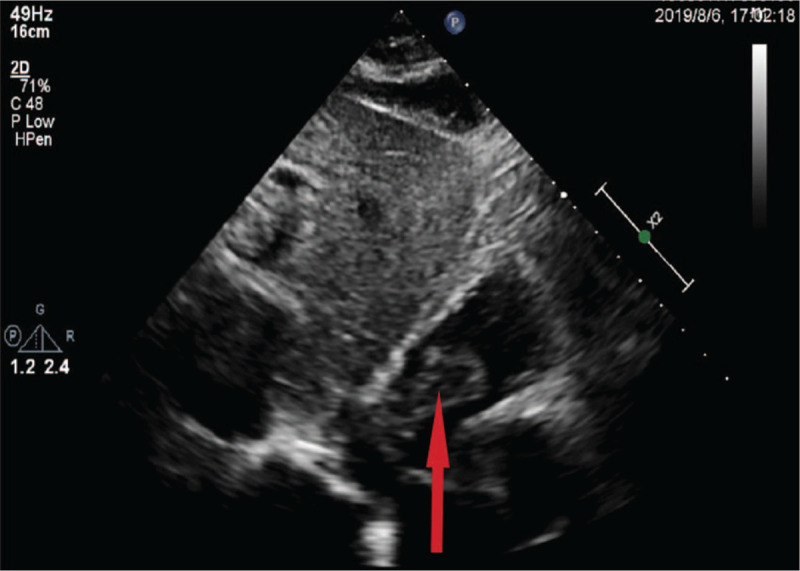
An echocardiogram showed an irregular mass shadow in the right atrium (↑).
